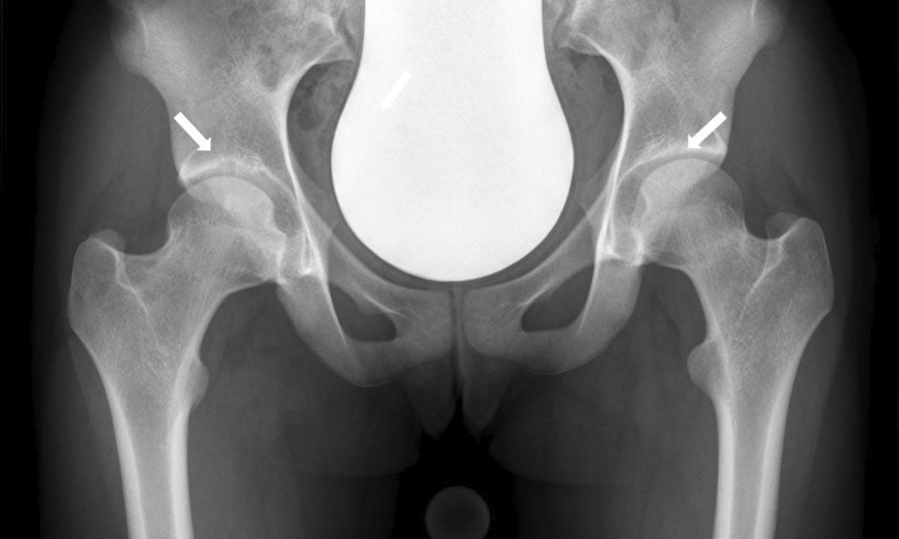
Radiograph of a 17-years-old female participant with SAF on both sides (white arrow)
